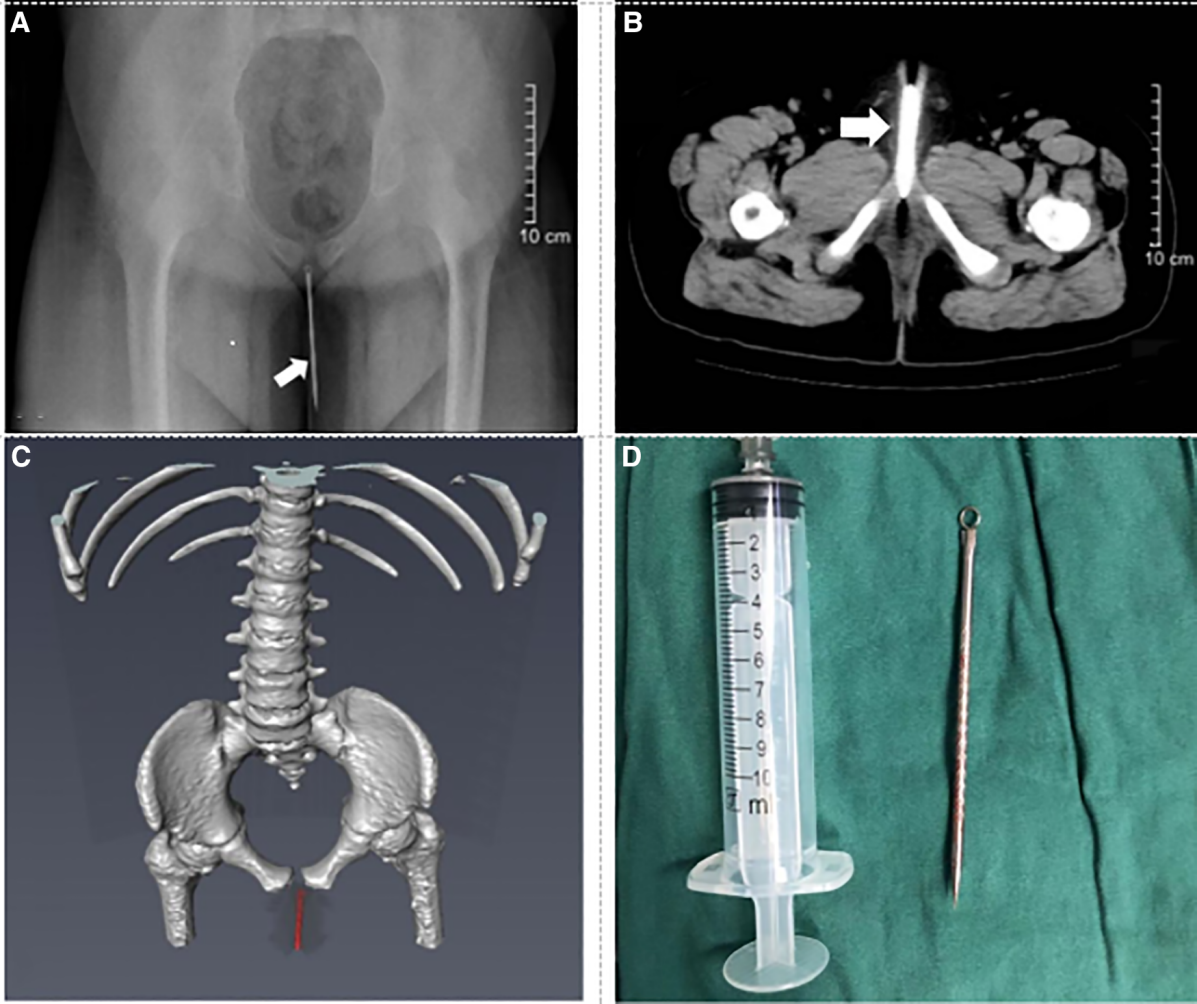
Preoperative imaging data and foreign body. (A) Plain film x-ray shows the urethral foreign body (see arrow). (B) CT of the pelvis shows the straight foreign body in the urethra (see arrow). (C) Three-dimensional reconstruction CT shows the straight foreign body in the urethra (red mark). (D) Foreign body is an acne needle about 10 cm long.
